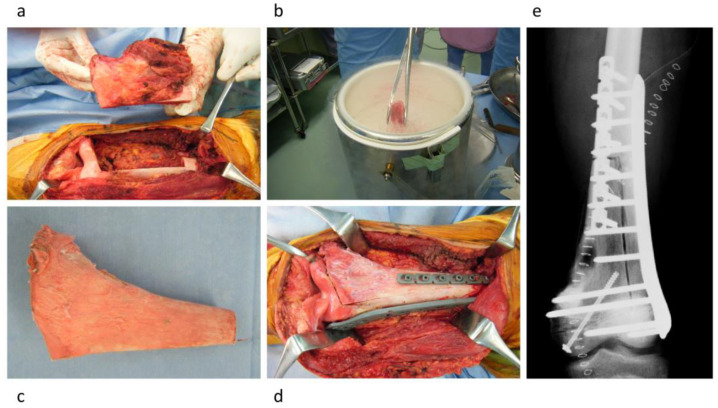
Hemicortical freezing procedure. (a) Hemicortical resection of the tumor. (b) Freezing in liquid nitrogen. (c) Tumor-bearing frozen bone. (d) The tumor-bearing frozen bone was used for reconstruction of the bone defect. (e) Postoperative X-ray.
